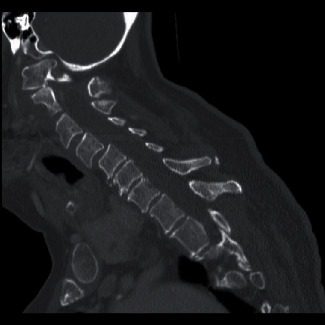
Cervical CT showing 1 of the multiple C1 fractures.
